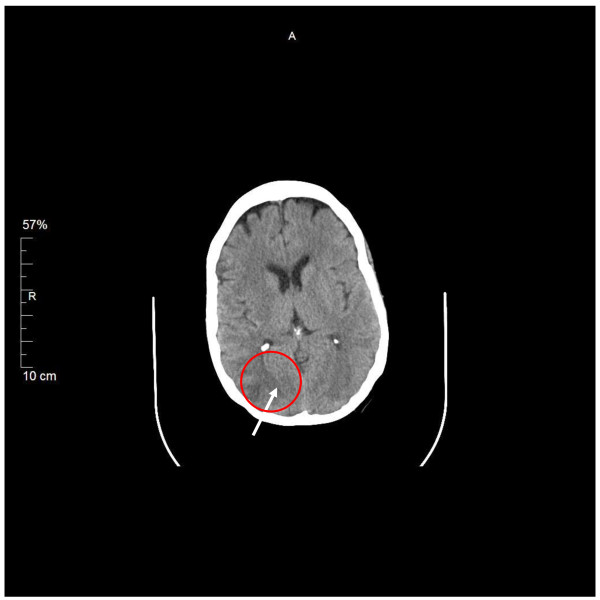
Computed tomographic image showing findings in the brain of a patient with posterior reversible encephalopathy syndrome. Subtle evidence of a possible acute abnormality in the right occipital lobe (arrow) in addition to an unusual pattern of calcification in the cerebral hemispheres.
